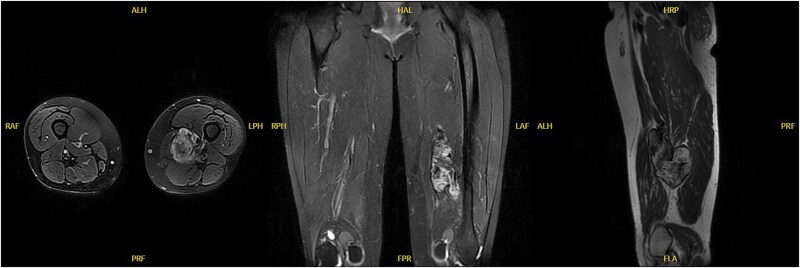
MRI revealed a lobulated mass.
